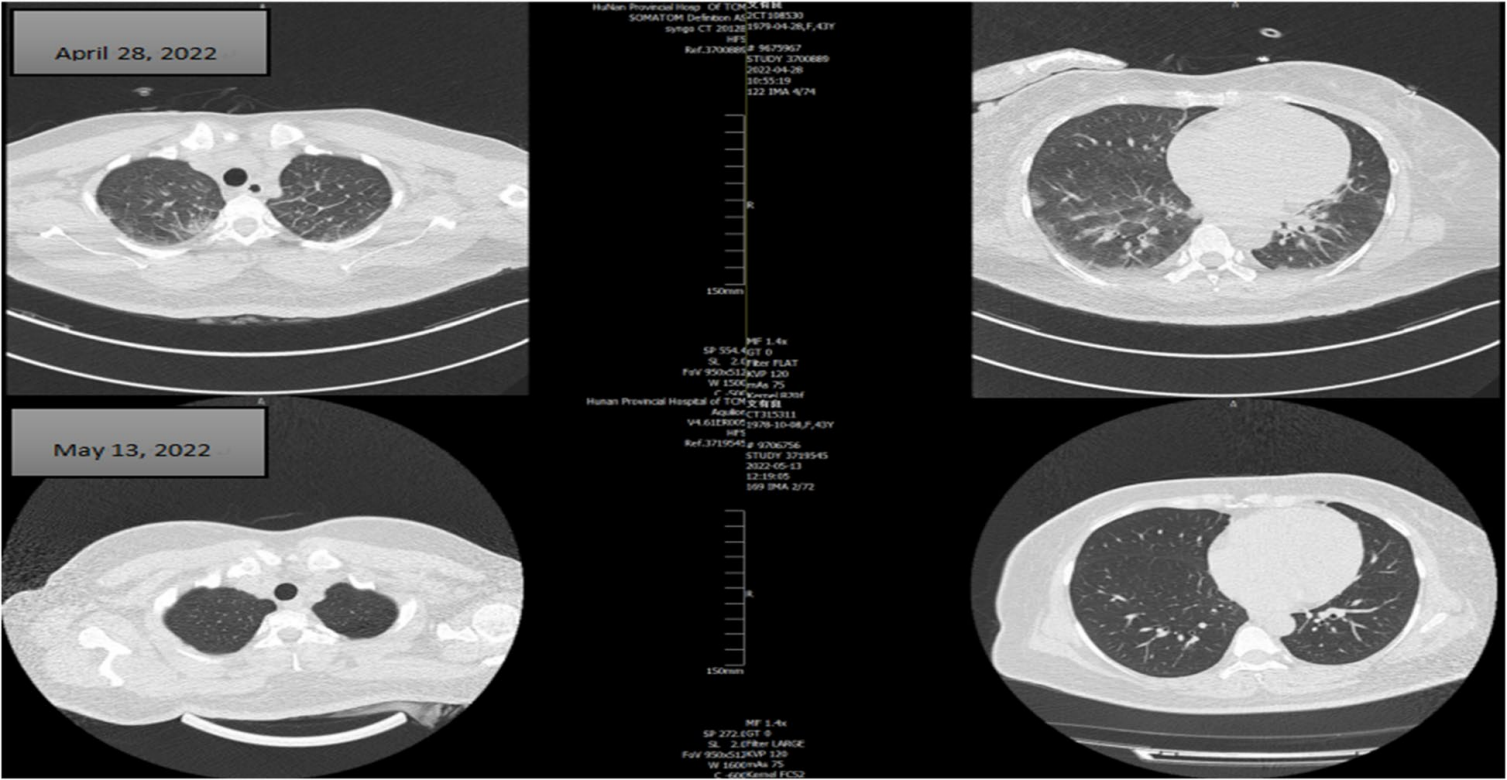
Lung CT on April 28, 2022, suggesting interstitial pulmonary edema; repeated lung CT on May 13, 2022 shows resolution of pulmonary edema
